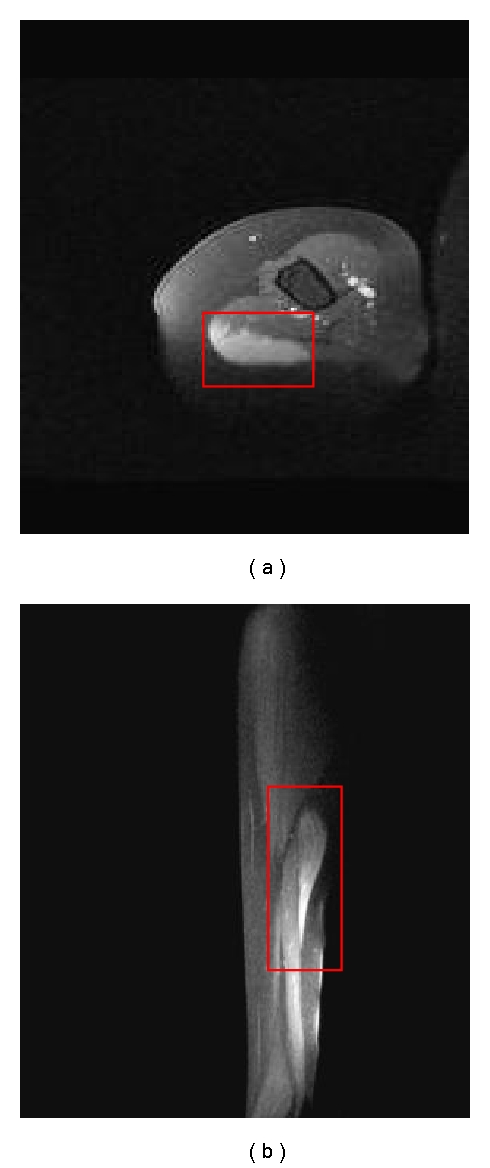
Pretreatment axial (a) and sagittal (b) postgadolinium contrast MRI of the right upper arm with enhancing mass in the posterior lateral aspect of the long head of the right triceps muscle outlined in red.
